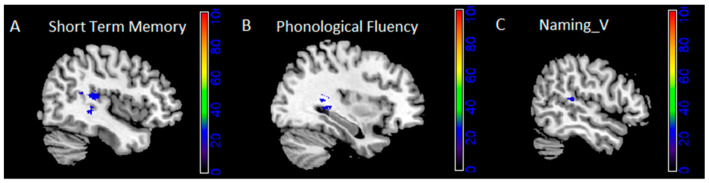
Subtraction of lesion masks of patients scoring pathologically from patients with normal performance (minimum 20% overlap) on the different tasks: short-term memory (A), phonological fluency (B) and verb naming (C) MR images are displayed following radiological convention (left is right and vice versa). V = verbs.
